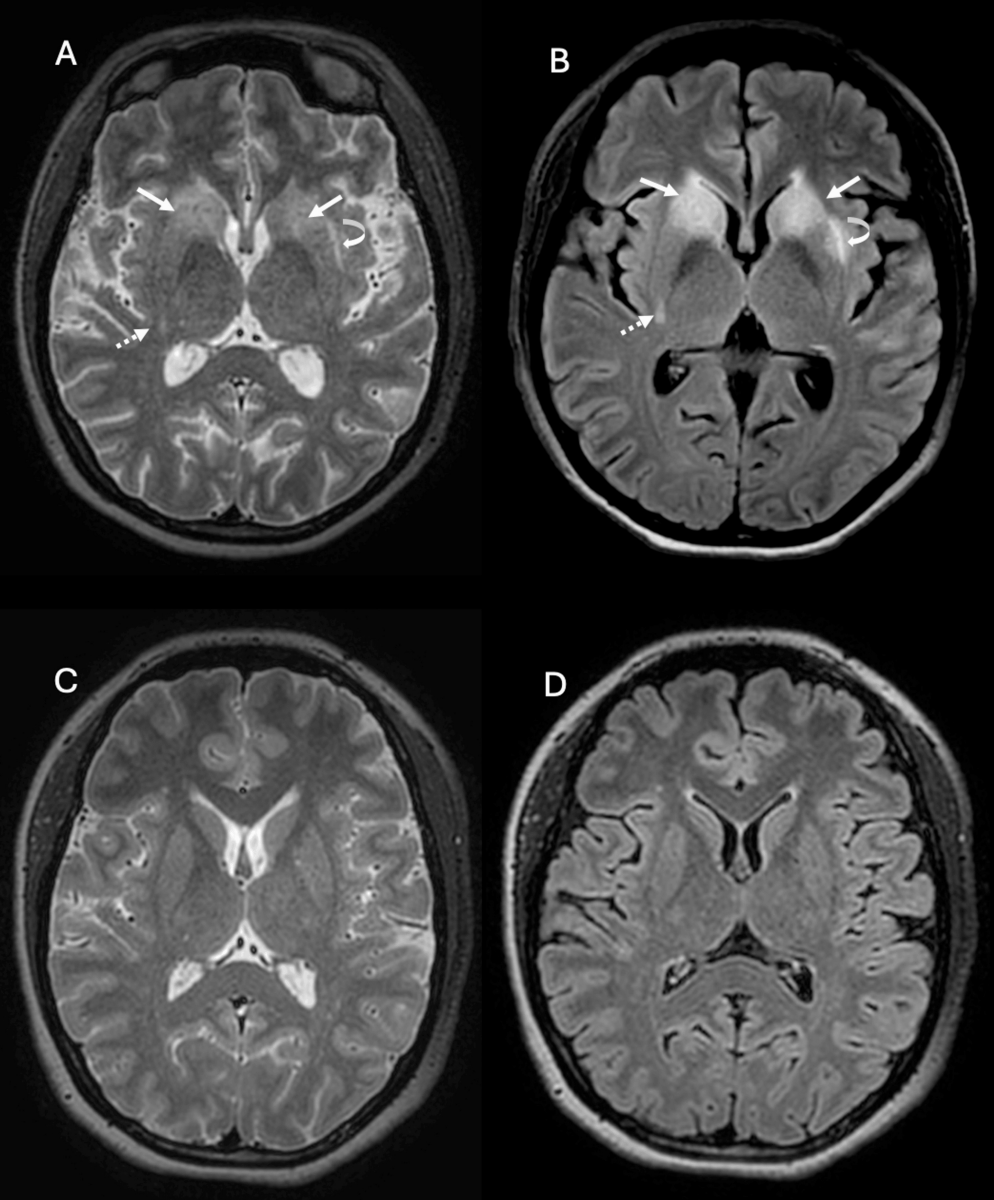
Axial MRI brain images in T2-weighted (A) and fluid-attenuated inversion recovery (FLAIR) (B) sequences showing hyperintense signals in both basal ganglia (arrow), left external capsule (curved arrow), and posterior right putamen (dashed arrow). Mild effacement of the frontal horn of both lateral ventricles is also noted. A follow-up study (C, D) reveals complete resolution of the hyperintense signals, indicating a favourable response to prompt and appropriate treatment.
